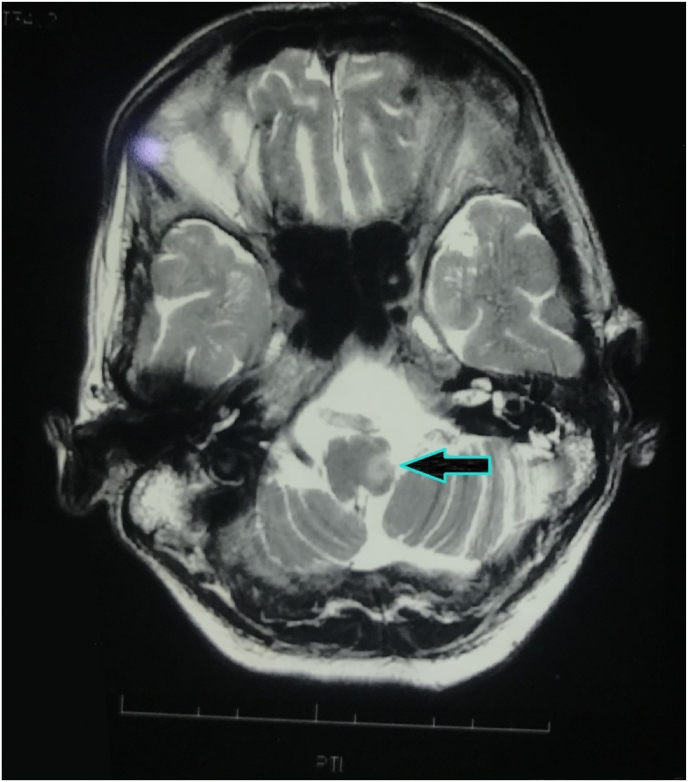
MRI Brain showing infarct in left lateral medulla hypodense area (pointing arrow) (without contrast).
